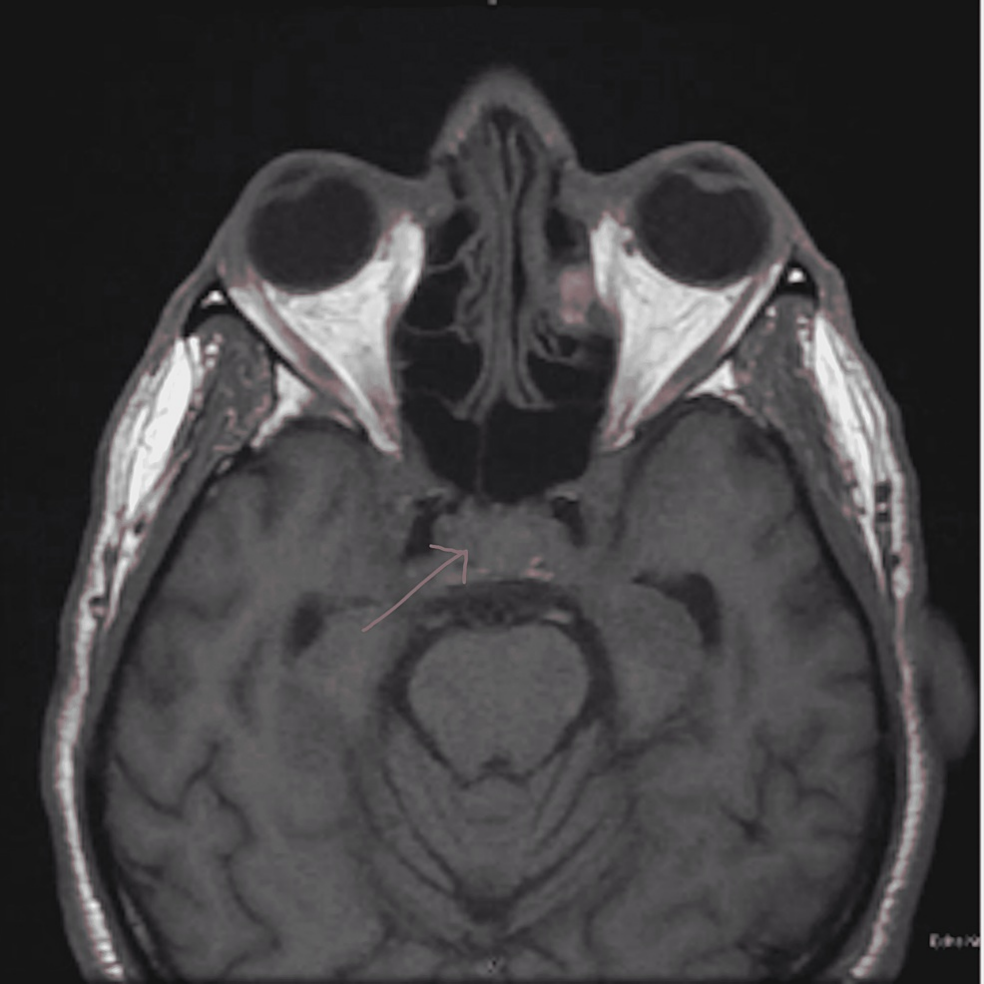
Pre-contrast T1 MRI Brain Demonstrating Diffuse Abnormal Thickening and Enhancement of the Cavernous Sinus
